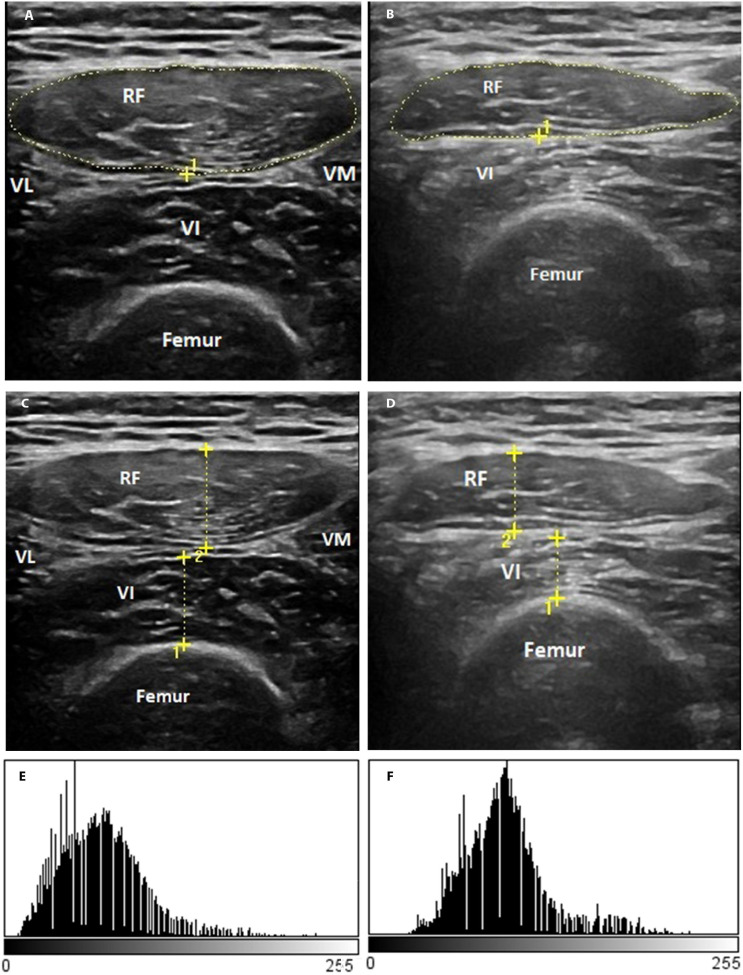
Representative qualitative muscle ultrasound image: rectus femoris cross-section area (A,B), the thickness of the anterior compartment of the quadriceps muscle (rectus femoris and vastus intermedius) (C,D), and rectus femoris echogenicity (E,F). The representative image is of a male patient, a 55-year-old man with a positive diagnosis for SARS-CoV-2 infection and that showed a severe illness.
